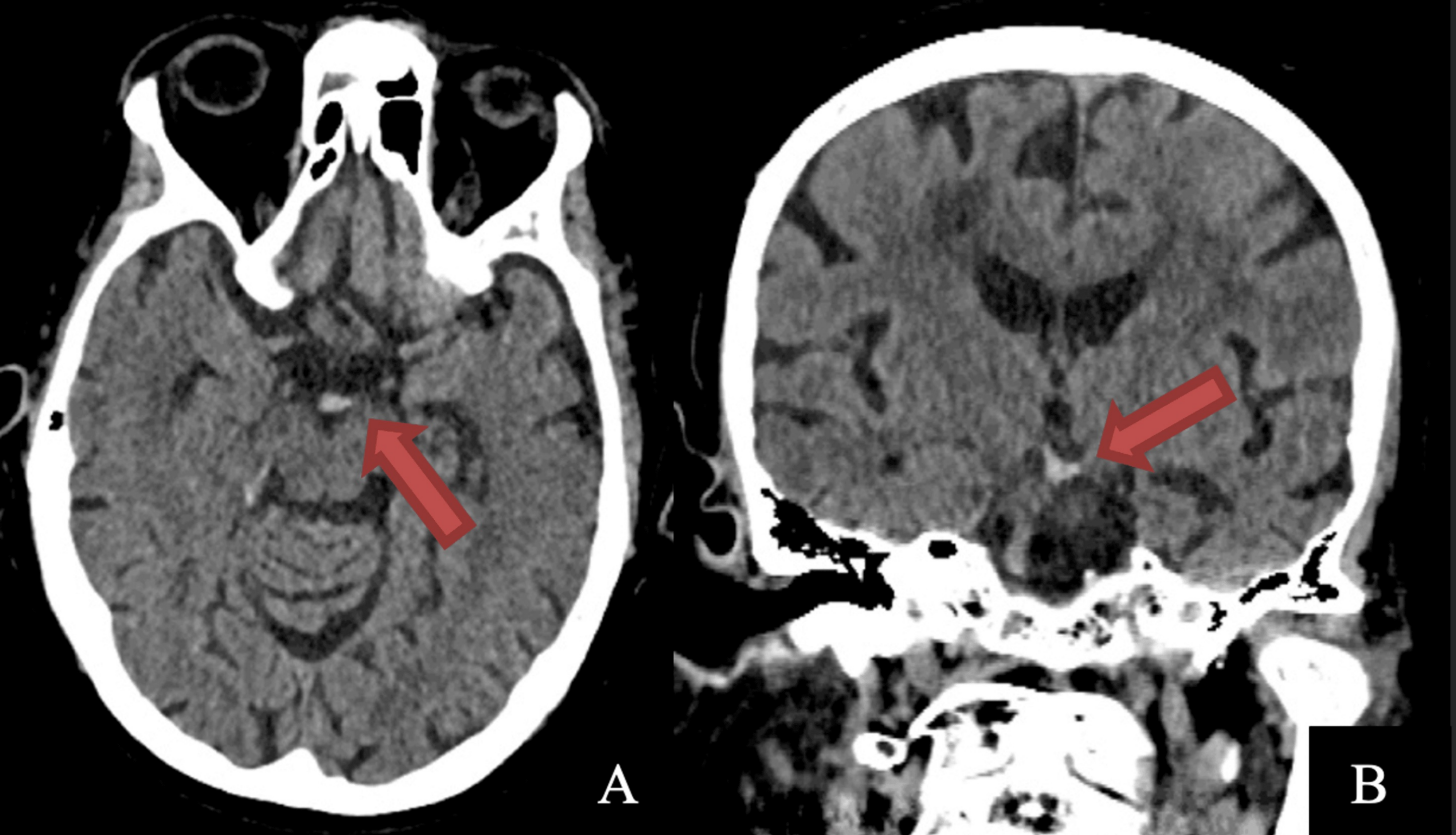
Preoperative brain CT scan of the patient (A) Axial image (B) Coronal image. A hyperdense basilar tip is evident in both images.
